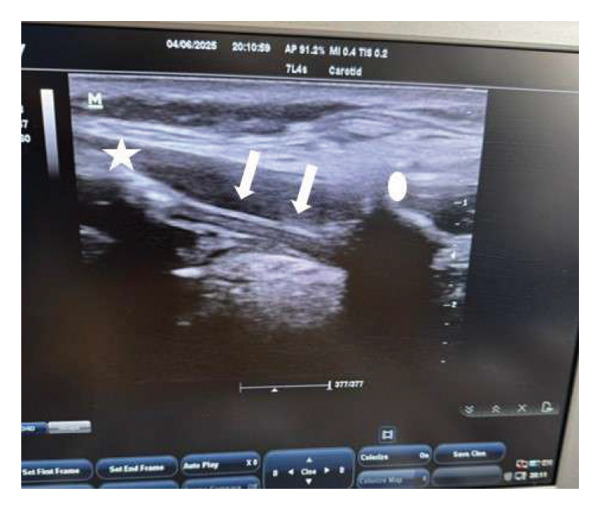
Superior laryngeal nerve block. An ultrasound image shows the anatomical landmarks for the injection site. The star sign indicates the hyoid bone, while the round sign indicates the thyroid cartilage. The arrows show the thyrohyoid membrane, which is the injection site.
